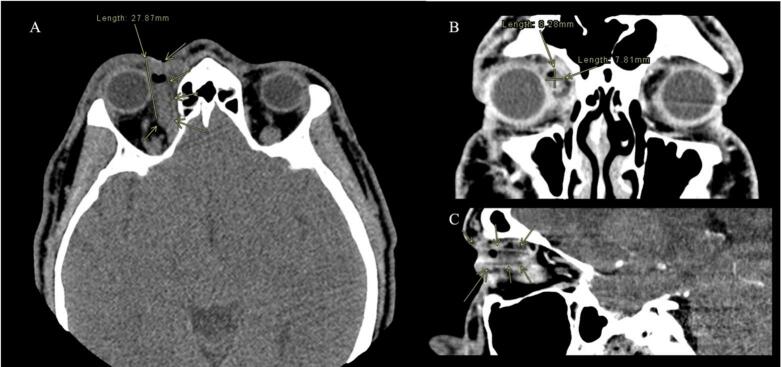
CT of the orbit demonstrating the penetrating straw. A) Axial view of orbit. Arrows pointing to tubular foreign body with a length of 27.87 mm. B) Coronal view of orbit. Arrows pointing to tubular foreign body with two diameter measurements of 8.28 mm and 7.81 mm. C) Sagittal view of orbit. Arrows pointing to tubular foreign body.
